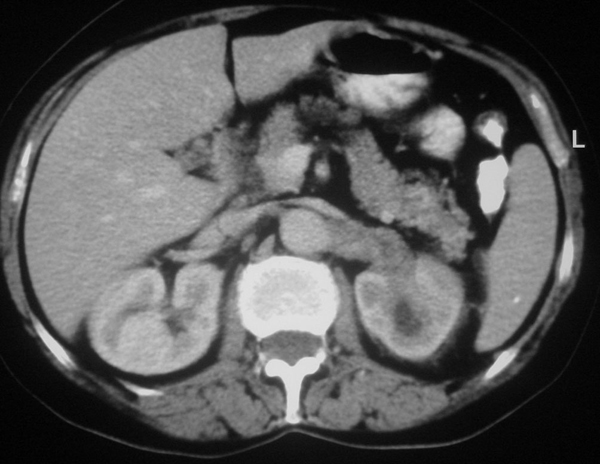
Axial post-contrast computed tomography scan, with normal cortical enhancement of the left kidney and the non-enhancing mass extending to the left para-aortic space along its vascular pedicle.
